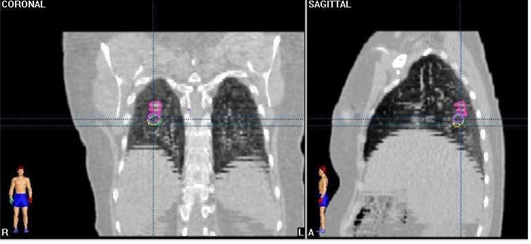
Reproducibility of tumor position during repeated DIBH CT scans fused on the FB CT scan (shown in pink).
